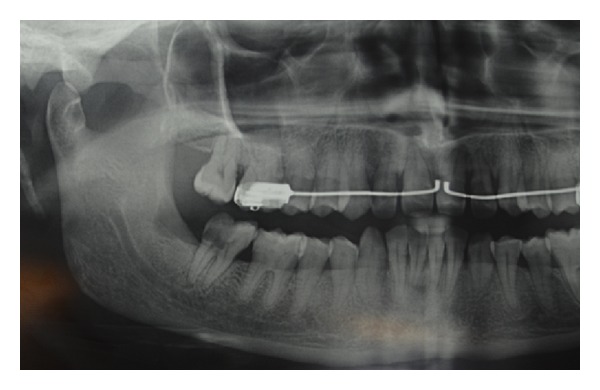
Preoperative radiographic appearance of radiolucent area back to tooth 47.
